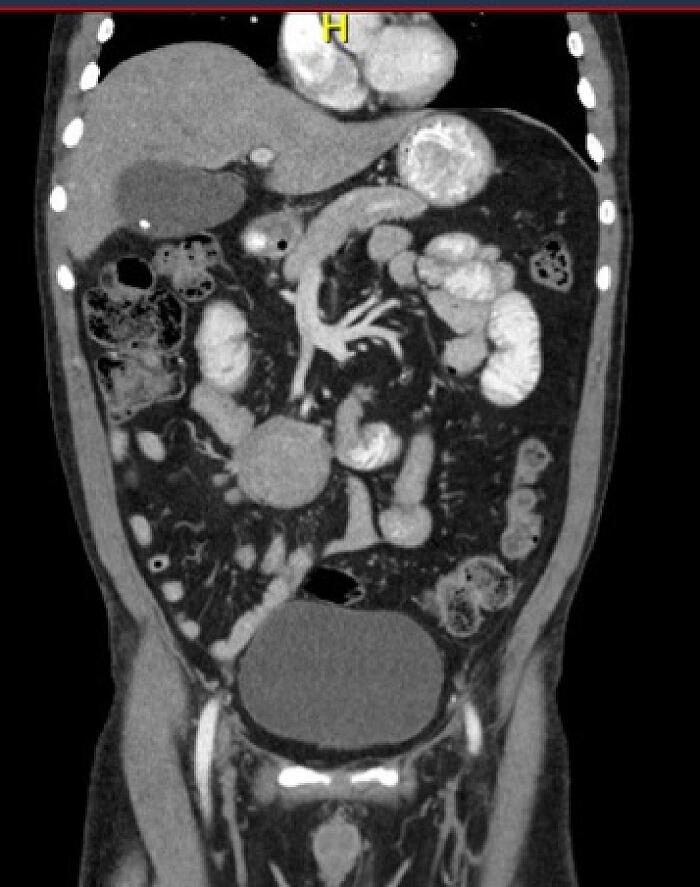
Coronal contrast-enhanced abdominal CT scan section, showing a round, homogeneous, solid mass in the mesogastrium, with compressive effect on the inferior vena cava (IVC).
